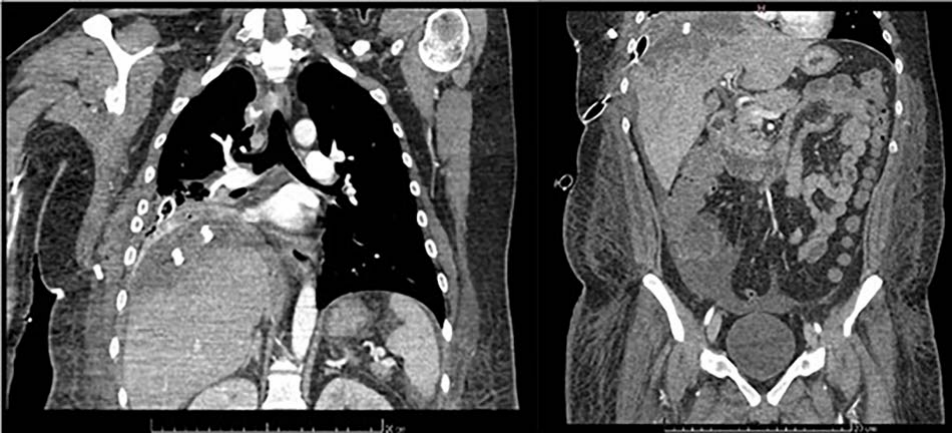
Frontal thoraco-abdominopelvic CT at 9 days after surgery: subpleural collection of 79 × 34 mm, heterogenous, hypodense hepatic lesion with contrast enhancement on its borders, fluid collection around the liver, paracolic gutters and pelvic cavity.
